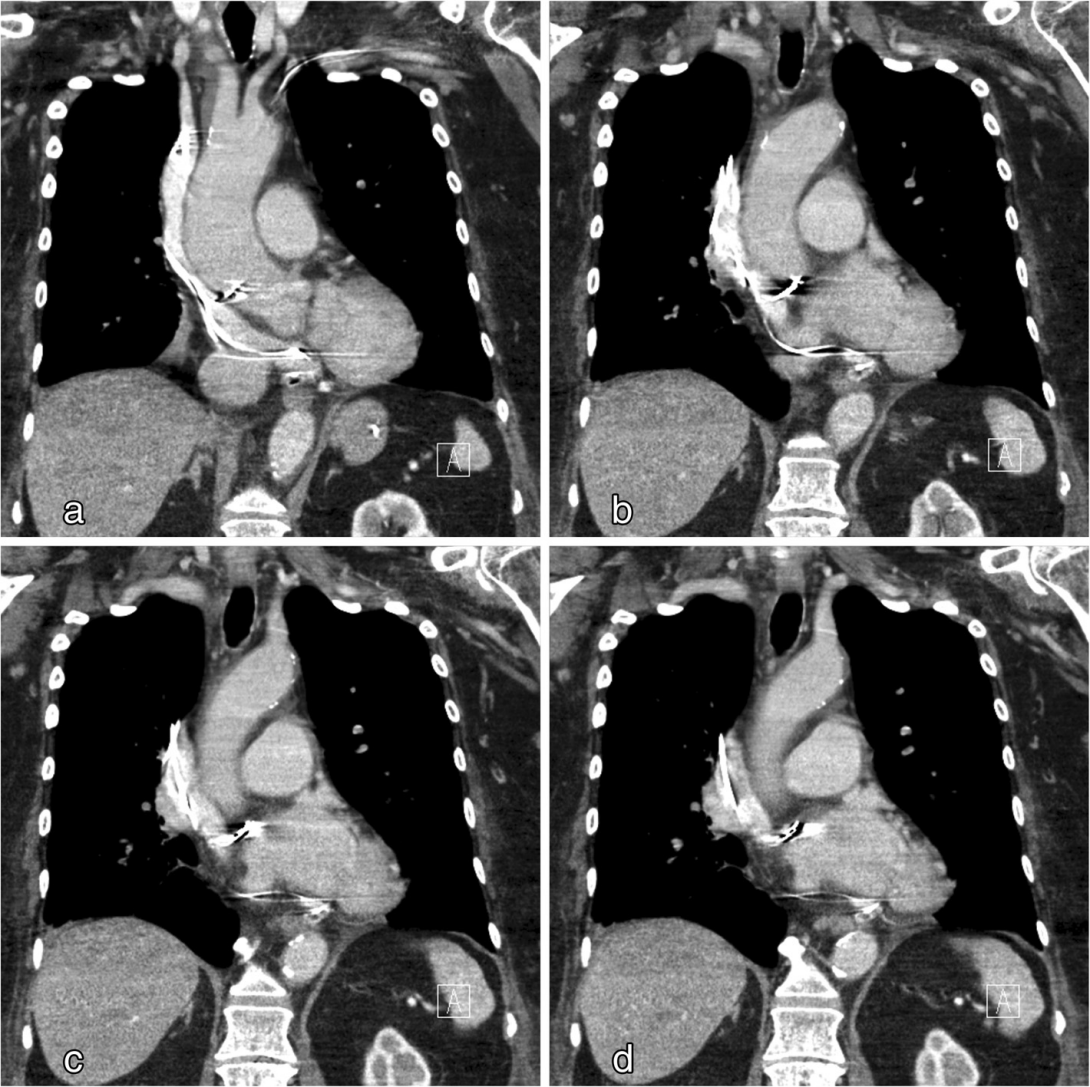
Computed tomography scan. The atrial lead connects to the roof of the left atrium; 2a-2d Four coronal CT slices, from ventral to dorsal.
